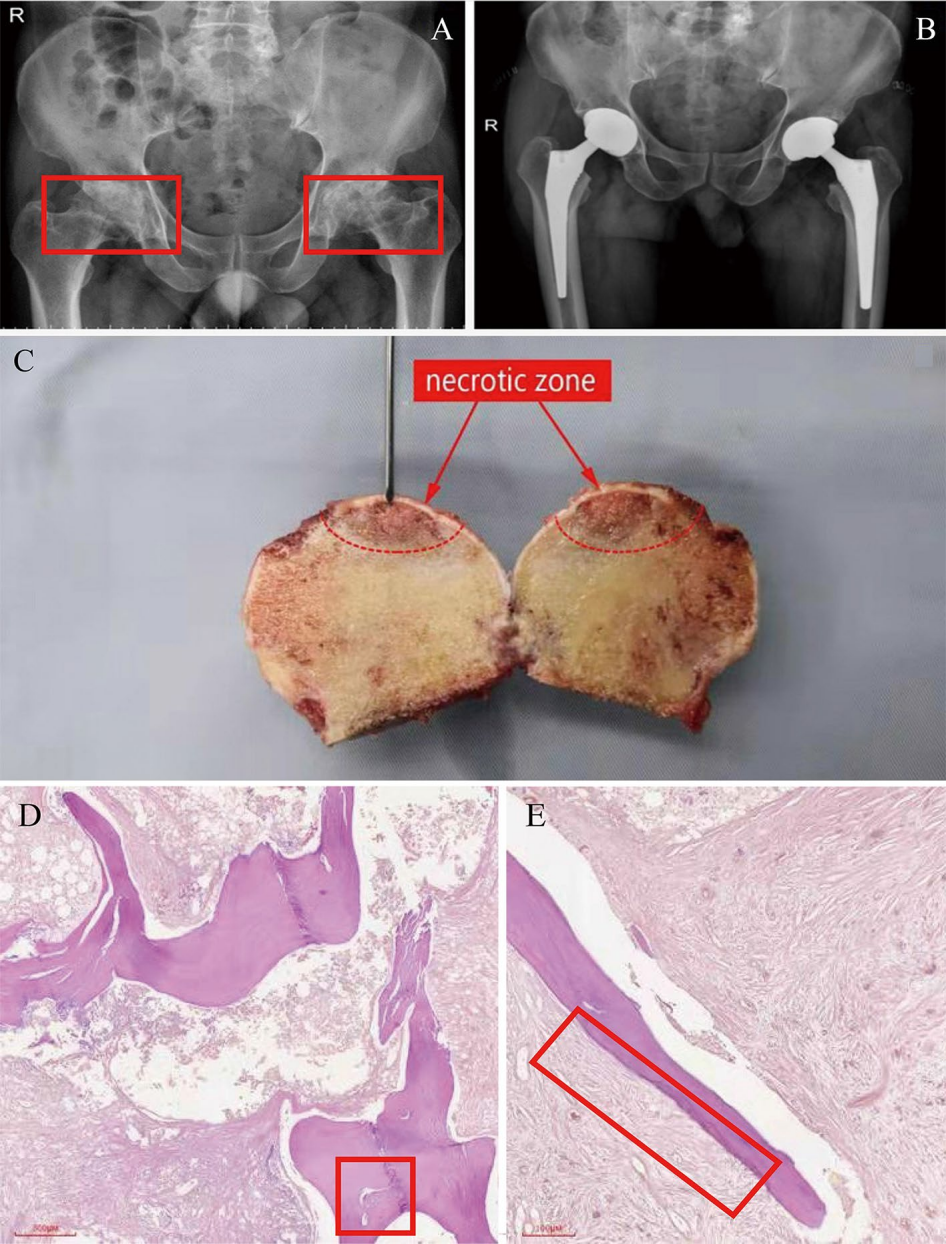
Imaging system for clinical diagnosis. (A) Preoperative pelvic frontal radiograph (X-ray). Bilateral femoral heads were flat, with multiple cystic low-density shadows and narrowed joint space (red boxes on both sides) (B) Implanted prosthesis on pelvis orthographic film (X-ray) (C) Diseased tissue (with a red arrow pointing to necrosis). (D) Morphological abnormality in bone trabecular (left red frame) (40 ×). (E) Bone trabecular necrosis (right red box) (100 ×).
